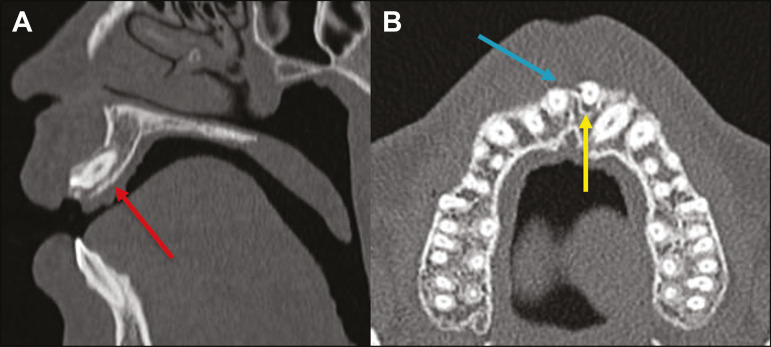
Sagittal and axial CT scans of the face (A and B, respectively) showing partial loss of the crown with root intrusion in the upper left lateral incisor (A) and a hypodense halo around the root of the upper left central incisor (B), with anterior dislocation of that tooth, suggestive of dislocation (yellow arrow), together with traces of fractures in the alveolar process in the maxila between the central incisor and the upper left lateral incisor (blue arrow).
